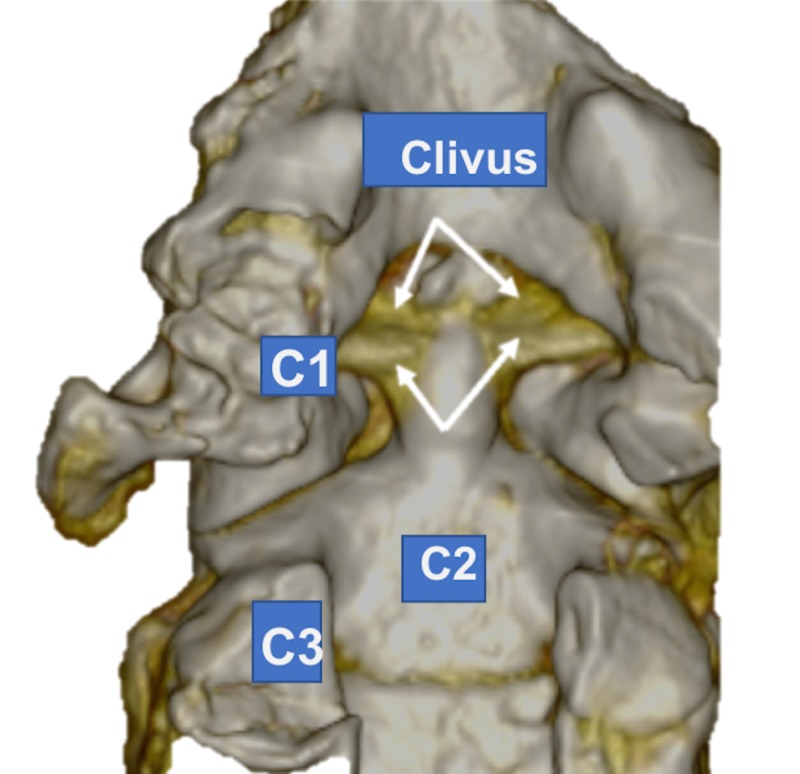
3D computed tomography (CT) reconstruction of the duplicated alar ligaments (arrows) as seen in the case reported here
